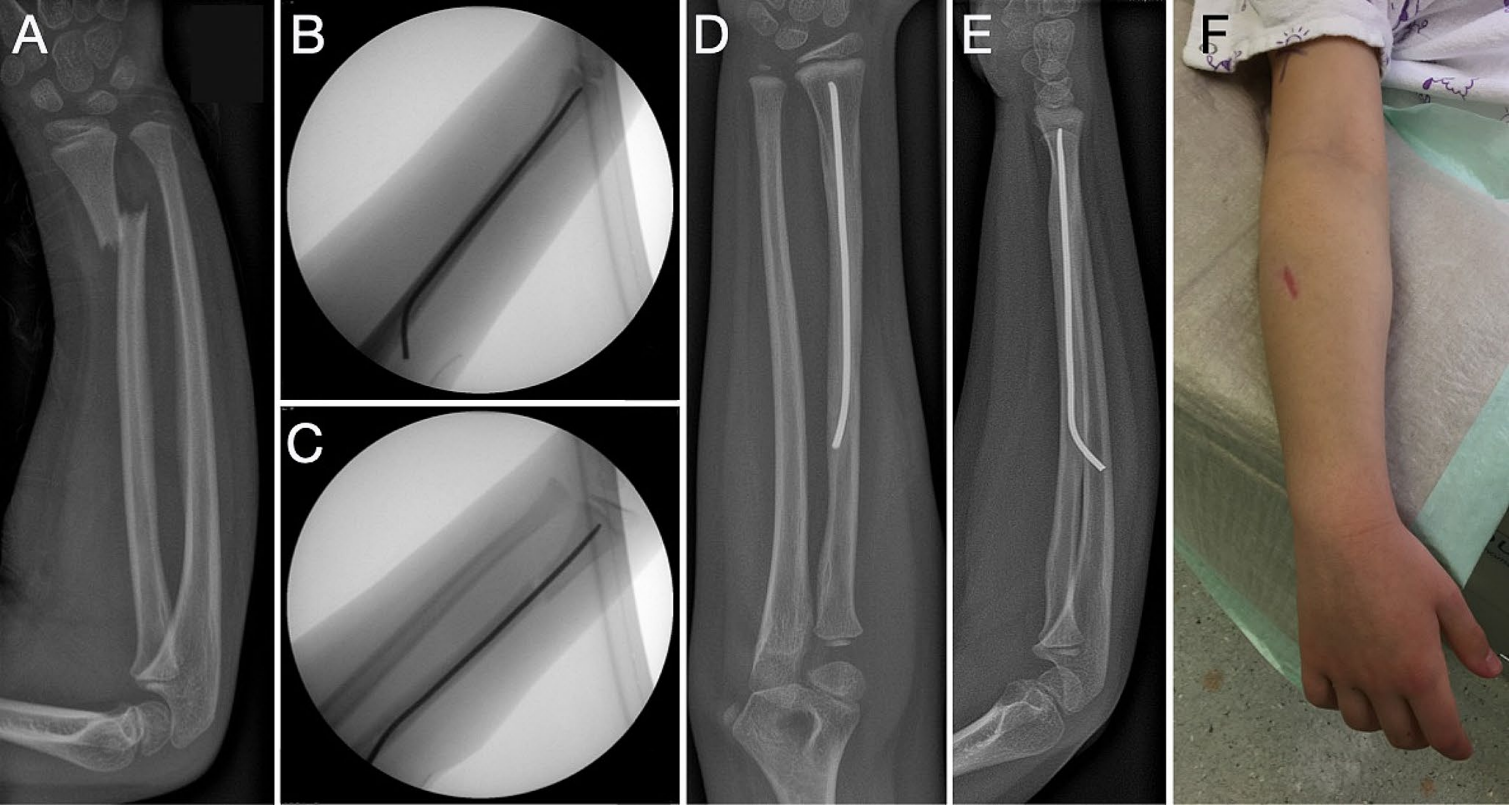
Implantation technique using Thompson’s approach. Implantation technique using Thompson’s approach in a displaced fracture located at the metaphyseal diaphyseal junction of the radius: X-ray at admission (A), during surgery (B, C) and before metal removal (D, E). Active scar at the dorsal aspect of the patient’s forearm following skin incision at the “soft spot” (F)
